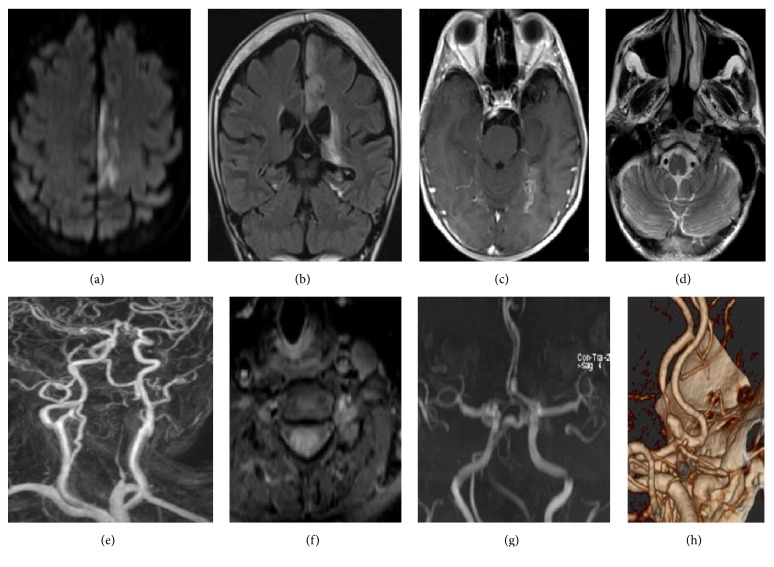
DWI showing (a) left acute anterior cerebral artery infarction and subacute posterior cerebral artery infarction in coronal FLAIR and (b) in postcontrast axial T1A (c); V4 segments of the vertebral arteries with a symmetrical appearance (d). Luminal narrowing along the left V2 in contrast-enhanced cervical MRA (e); hyperintensity in left V2 suggestive of intraluminal or intramural thrombus in fat-suppressed T1A (f); dissection presenting with narrowing and dilatation at the proximal part of the left A2 at 3D-TOF MRA (g) and volume rendering CTA (h). CTA was considered to be suggestive of dissected left V2, and the hyperintensity in T1A images was explained on the basis of intramural thrombus formation.
